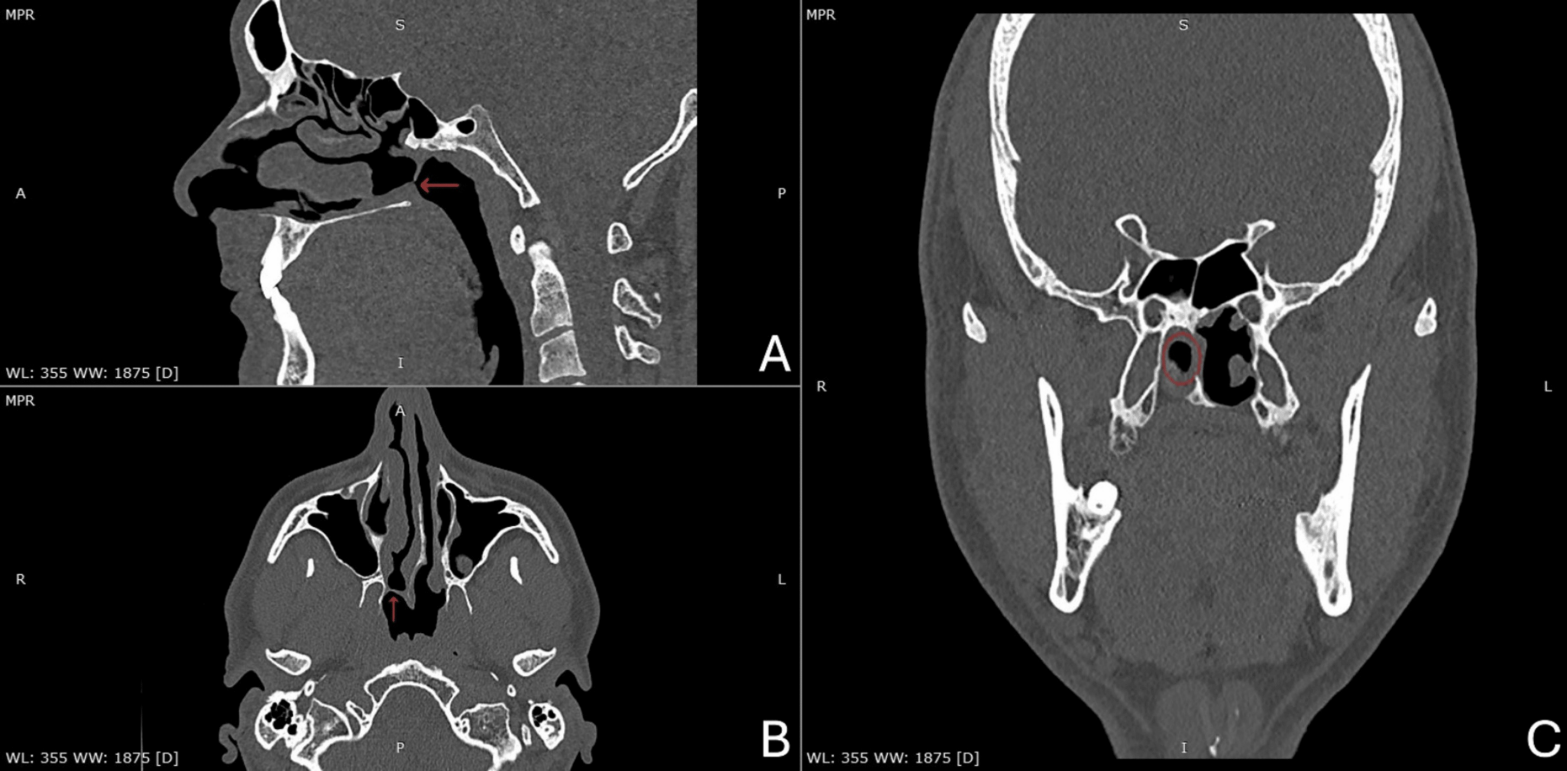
Multiplanar view of sinus CT scan A: Sagittal view. Near-complete obstruction of the right posterior choana from membranous tissue (red arrow). B: Axial view. Near-complete obstruction of the right posterior choana from membranous tissue (red arrow). C: Coronal view. Near-complete obstruction of the right posterior choana from membranous tissue (red eclipse).
